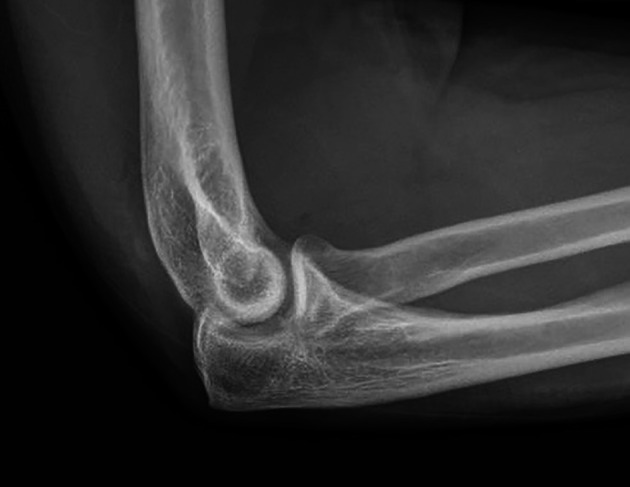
Postoperative anteroposterior and lateral radiographs confirming maintenance of anatomic reduction and stable fixation of the capitellar osteochondral fragment.
